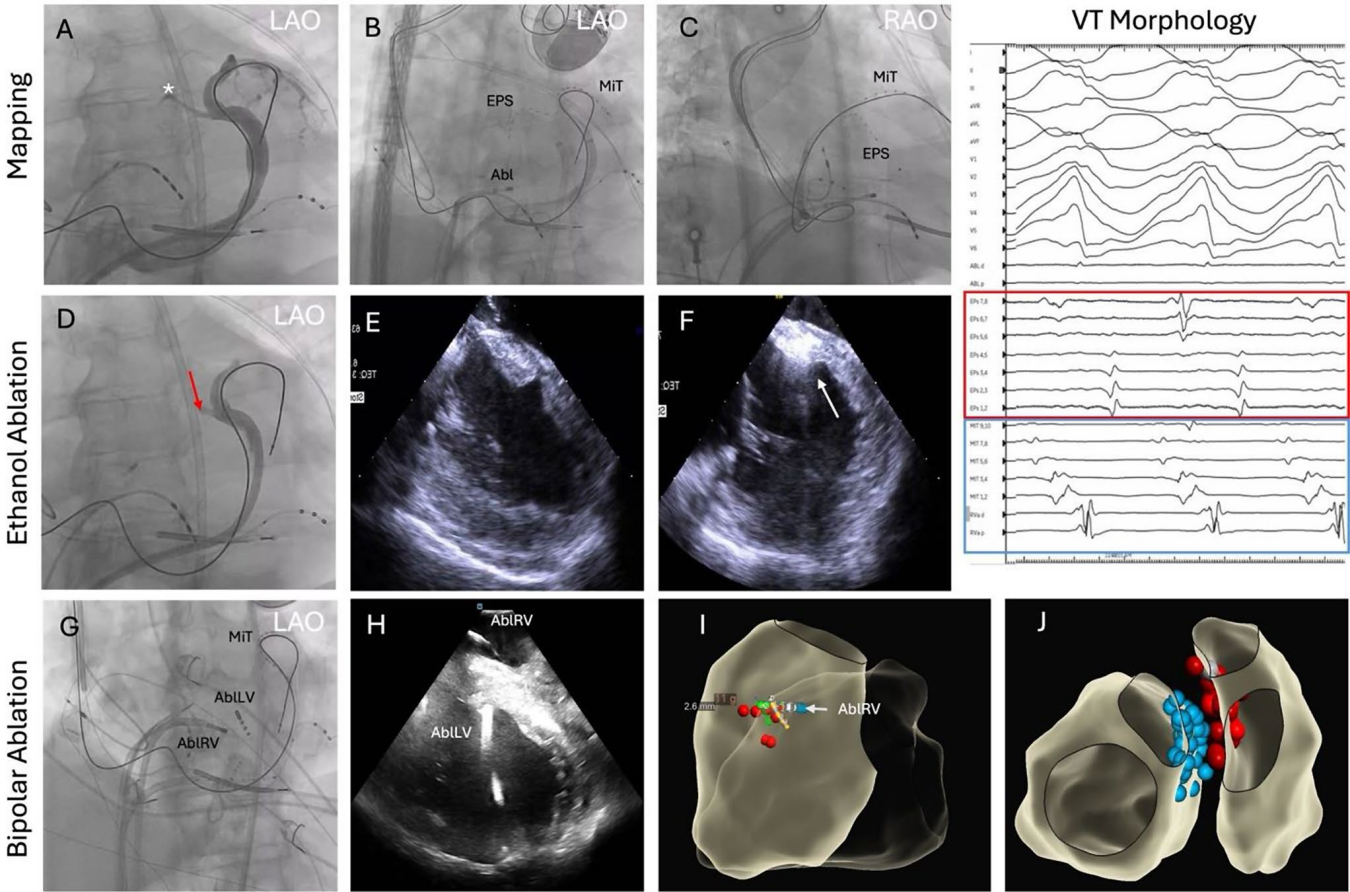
Septal ventricular tachycardia with RBBB pattern and right inferior axis (inset panel). After the anatomy of the coronary venous system was defined with venography (A), a Map-iT (MiT) catheter was placed in the AIV and an EPstar (EPS) catheter was placed in the first septal perforator vein (*) as seen in LAO (B) and RAO projections (C). Electrograms from both catheters can be seen on the VT morphology panel during arrhythmia, with mid-diastolic activity recorded by the EPstar catheter (red box). After failed endocardial ablation from both sides of the septum, ethanol ablation through the septal vein was performed (D; red arrow), with acute suppression but VT recurrence over the next days. Lesion formation can be seen on ICE with pre- (E) and post-ethanol infusion images demonstrating echodense lesion formation (F, white arrow). Ultimately, the patient was taken back to the lab and bipolar ablation was performed with two ablation catheters on opposite sides of the interventricular septum (AblRV and AblLV) as seen on fluoroscopy (G), ICE (H), and electroanatomic map (I). The final lesion set is demonstrated in panel J. AIV = anterior intraventricular vein, LAO = left anterior oblique, RAO = right anterior oblique, VT = ventricular tachycardia, ICE = intracardiac echocardiography
